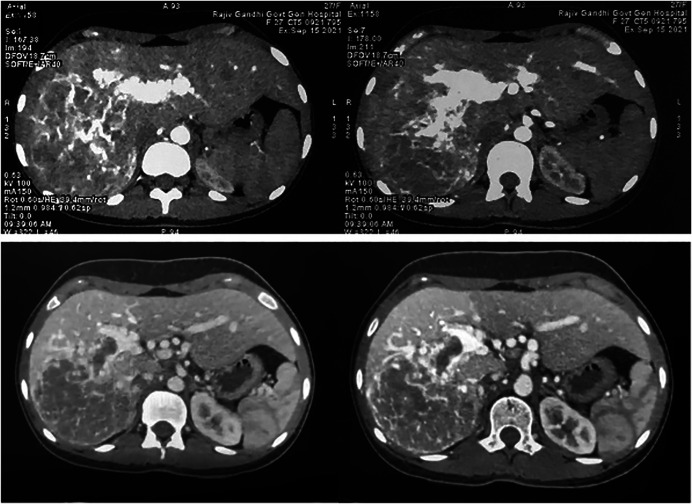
Multidetector computed tomography axial sections showing a heterogeneously enhancing mass lesion involving the right hemiliver with early arterial enhancement, persisting in subsequent phases, with prominent feeding right hepatic artery and right portal vein thrombosis.
